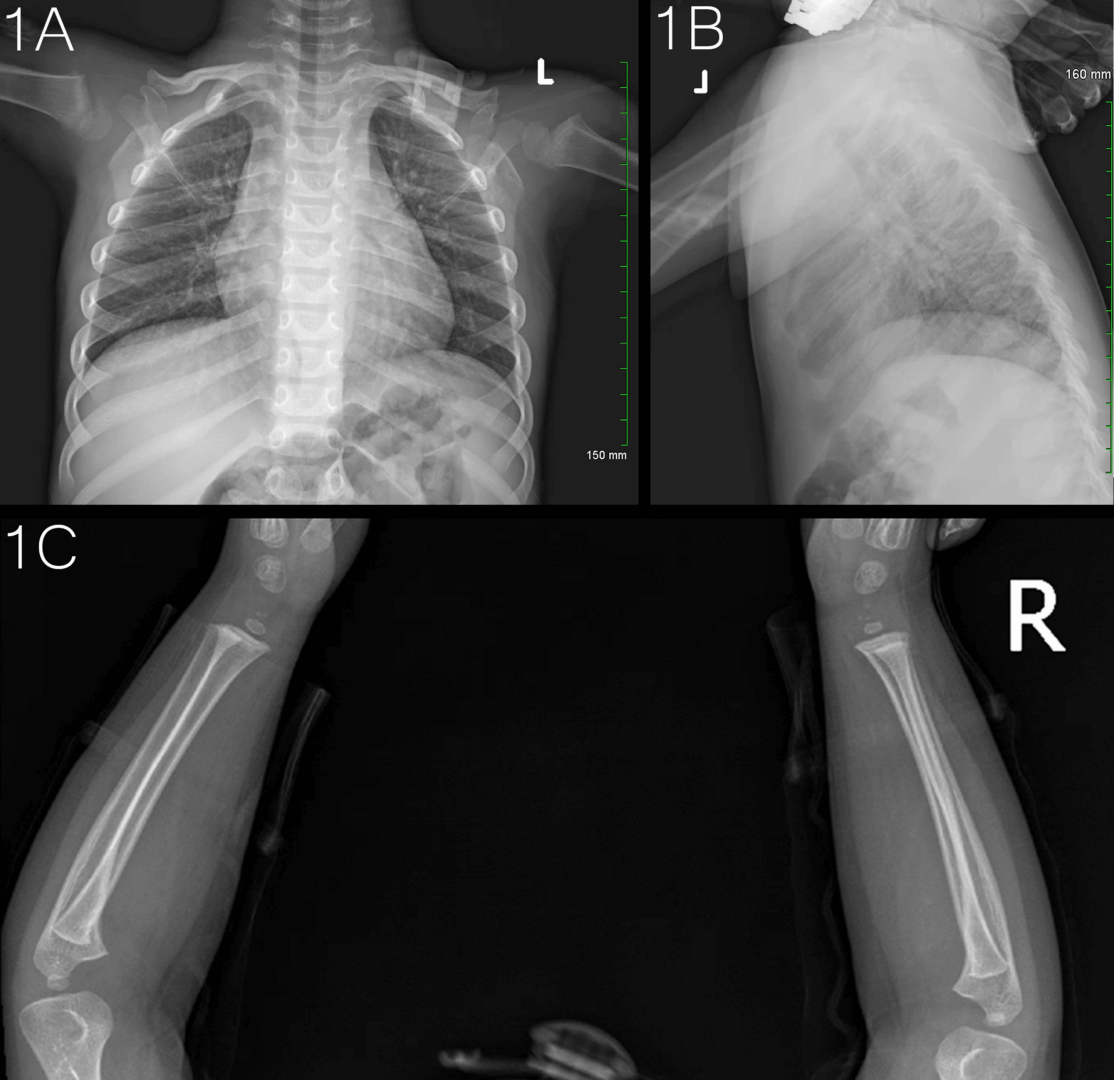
Chest and forearm plain radiographs. Chest AP view (1A, top left); chest lateral view (1B, top right); forearms lateral view (1C, bottom center).
